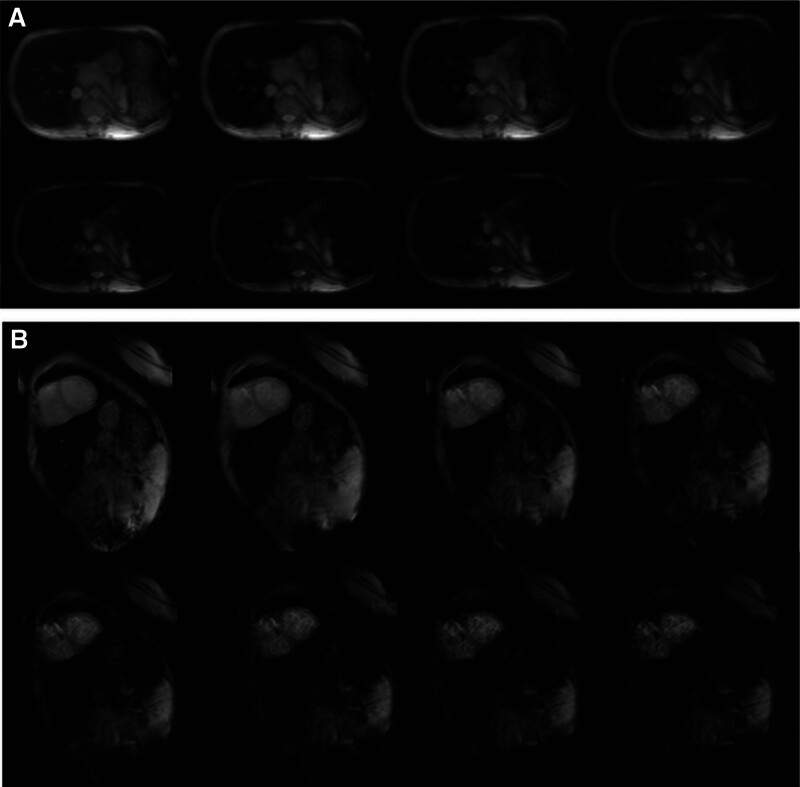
Magnetic resonance imaging (MRI) T2* measurements of (A) liver and (B) heart in the proband.
